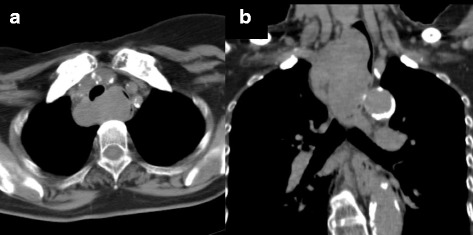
Computed tomography (CT). a, b A chest CT showed a lobulated mediastinum tumor extending to the cervix
